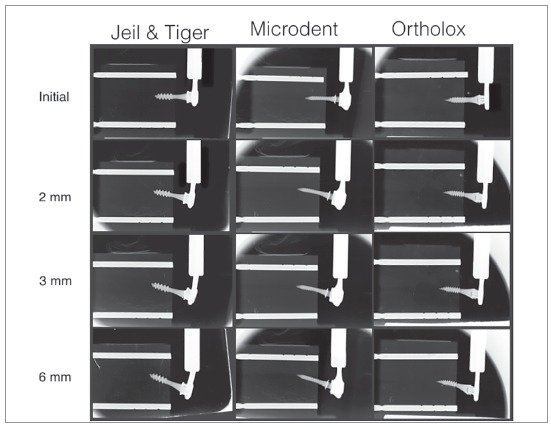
X-ray sequence (initial, 2 mm, 3 mm and 6 mm) of Jeil & Tiger, Microdent and Ortholox (left to the right) with double fastening wire.
